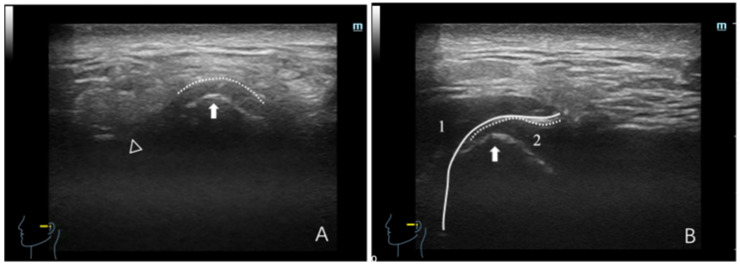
Ultrasonographic image depicting a normal TMJ. (A) Maximum intercuspal position; (B) full opening position. In panel (A), the dotted line denotes the joint capsule, depicted as a hyperechoic (white) line. The white triangle indicates the articular eminence, whereas the white arrow indicates the condyle, with the condyle surface manifesting as a hyperechoic line. In panel (B), (1) denotes the masseter, depicted as hypo-to-isoechoic, with white lines outlining the masseter boundary. (2) denotes the articular disc, which appears as a thin hypo-to-isoechoic band, with the dotted line delineating the joint capsule.
